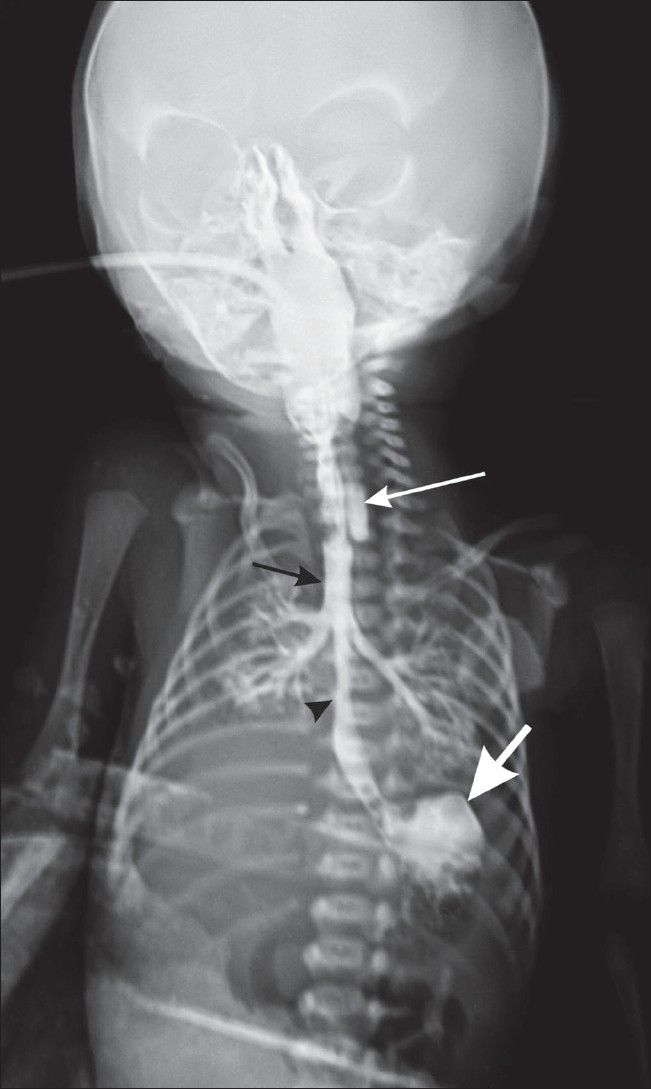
Chest radiograph of the baby with contrast shows the blindending upper end of the esophagus (long white arrow), contrast in the trachea (black arrow), the lower end of the esophagus communicating with the trachea (black arrow head) and contrast in the stomach (small, thick white arrow)
